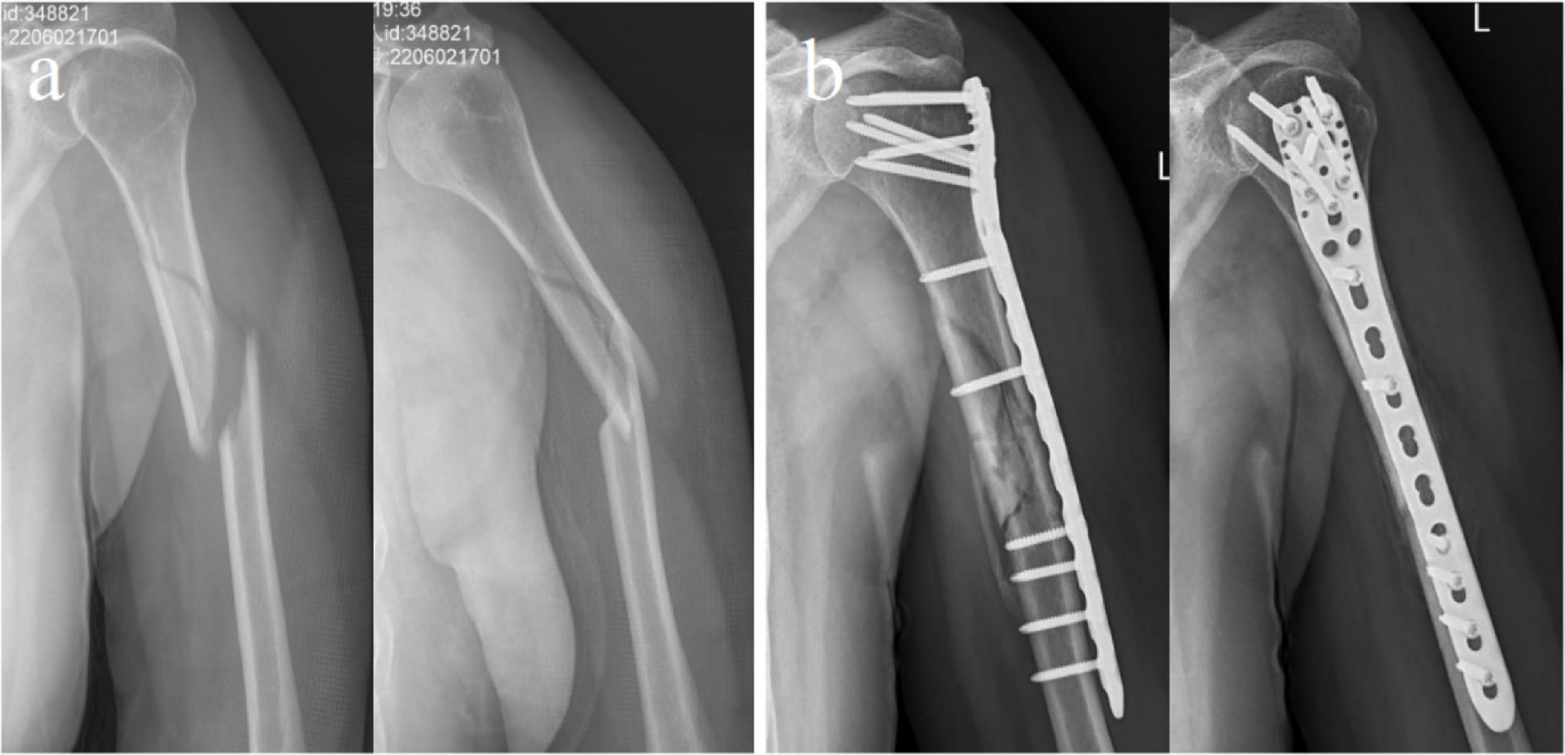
Case 1: a 64-year-old female, the AO/OTA classification was 12C1. a Preoperative X-ray radiograph of the middle-proximal humerus fractures. b The X-ray radiograph of full-length humerus 4 months after ORIF
